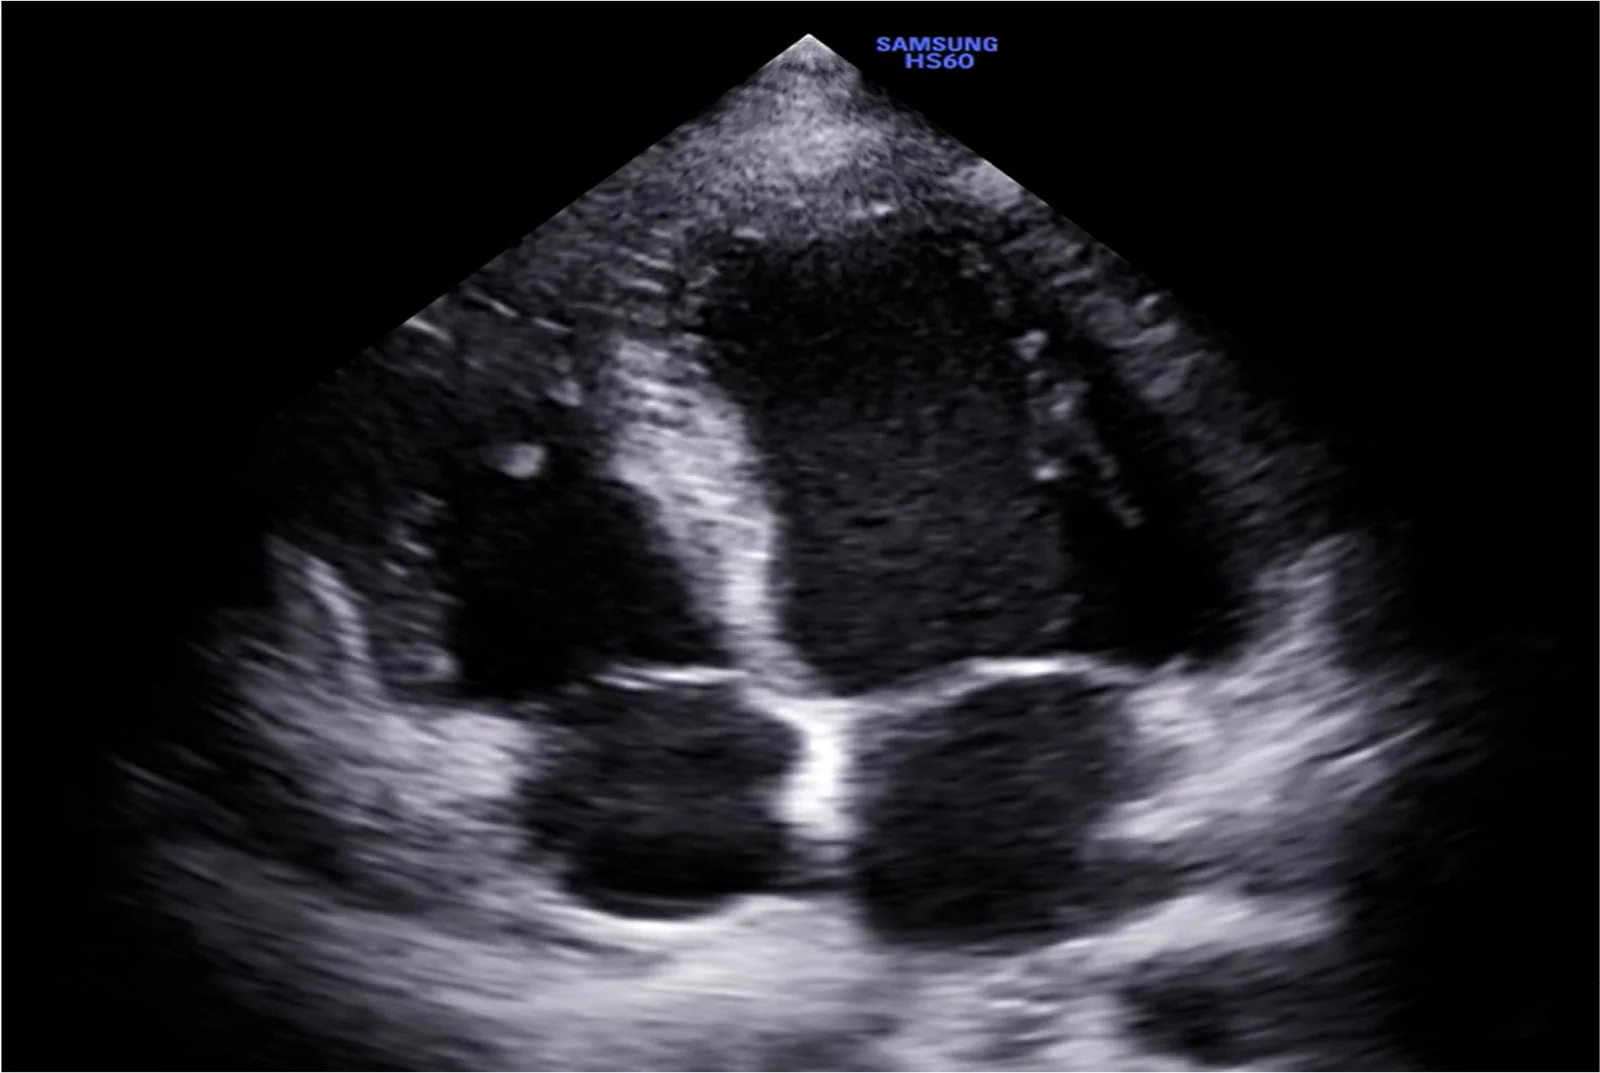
Follow-up transthoracic echocardiography (apical 4-chamber view) performed at 3 months showing complete resolution of the previously identified right atrial thrombus, with no residual intracavitary mass.
